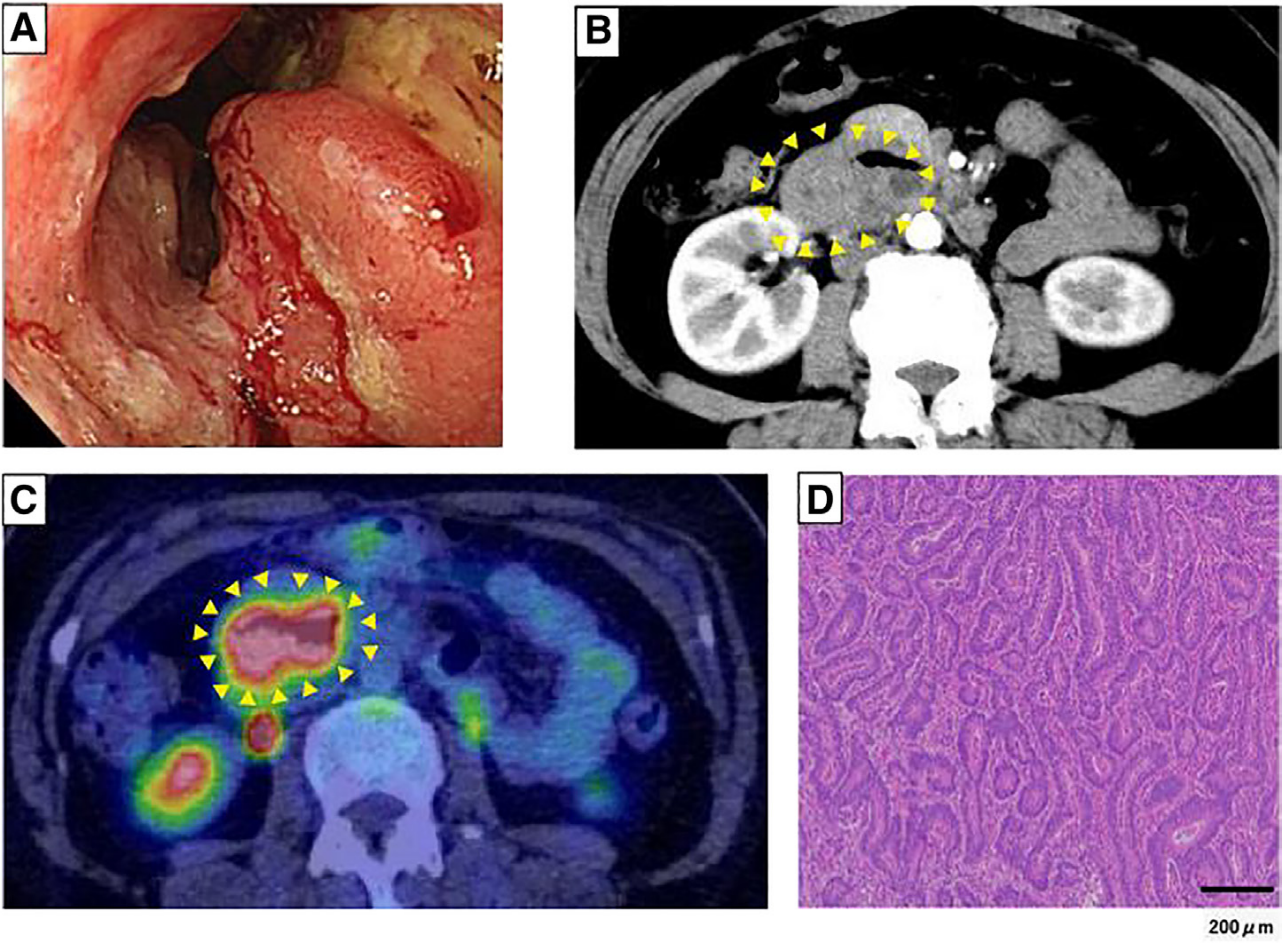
Esophagogastroduodenoscopy revealed an ulcerative lesion in the 3rd portion of the duodenum (A). CT showed duodenal wall thickening (arrowheads) without nodal or distant metastases (B). PET-CT demonstrated uptake in the descending duodenum (SUV_max_ 11.4) (arrowheads) without spread (C). Biopsy confirmed adenocarcinoma (D; scale bar: 200 μm). SUV_max_, maximum standard uptake value
